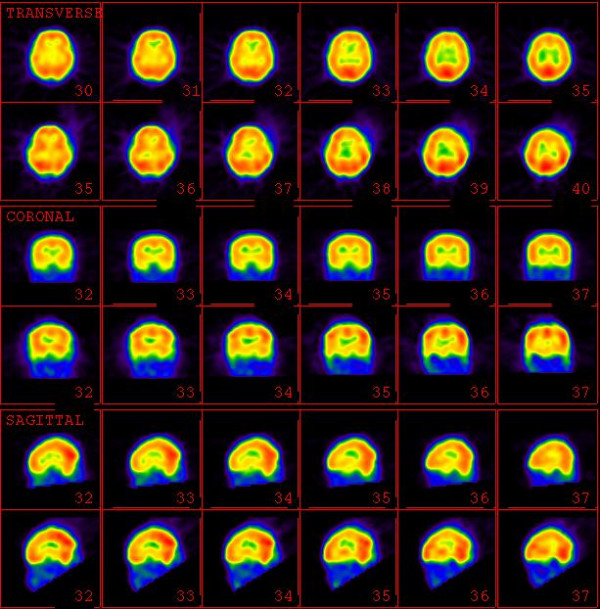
Pre-stimulation (upper rows) and Post-stimulation (lower rows) SPECT images of a volunteer control.
